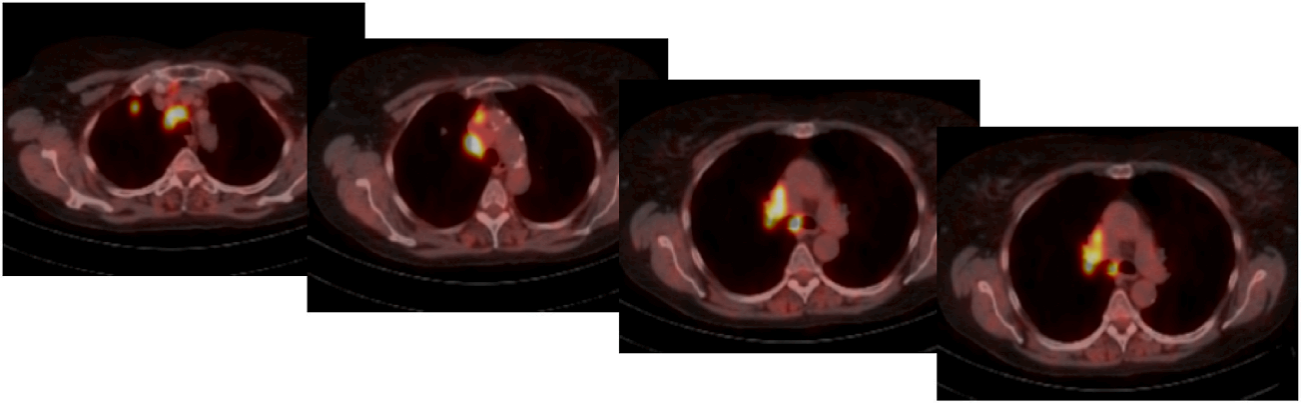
Initial PET-CT-Scan: Marked FDG-uptake of the primary lesion and mediastinal and hilar lymph nodes (N2, Robinson 3).
